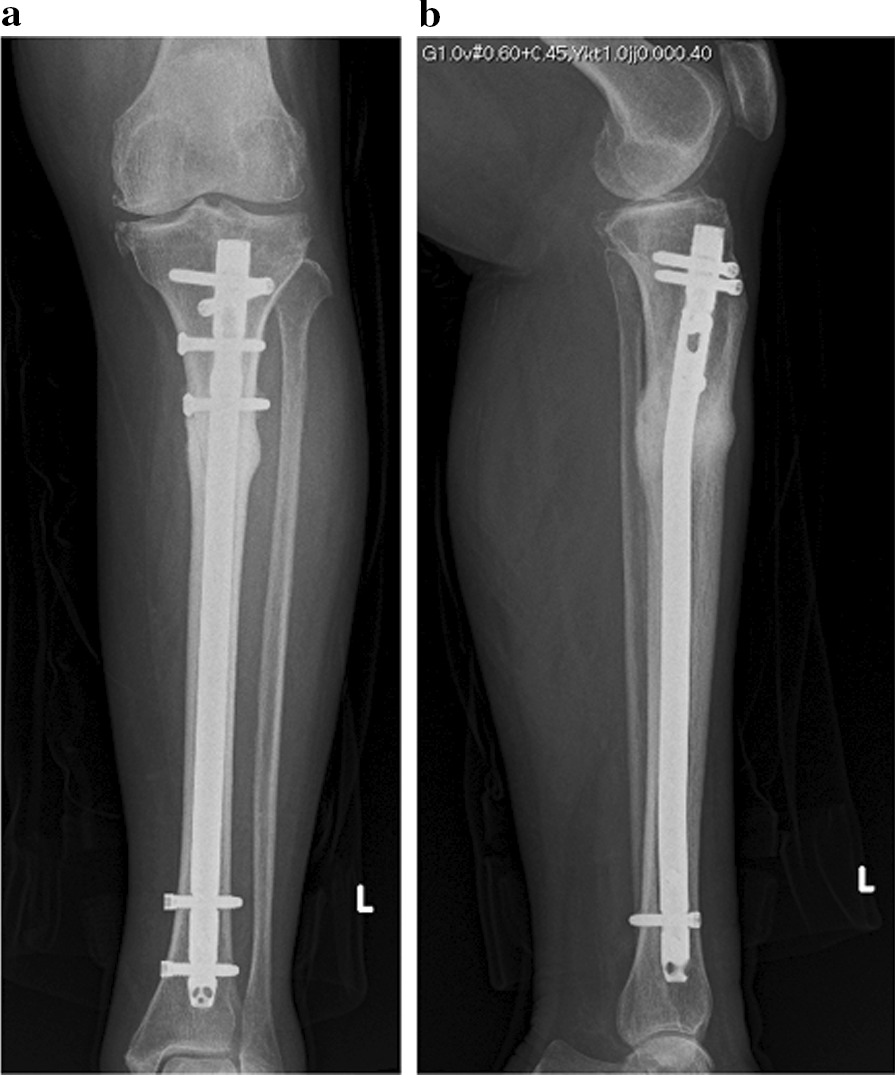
Two-directional X-ray image of her lower leg 4 months after surgery. The fracture was united
